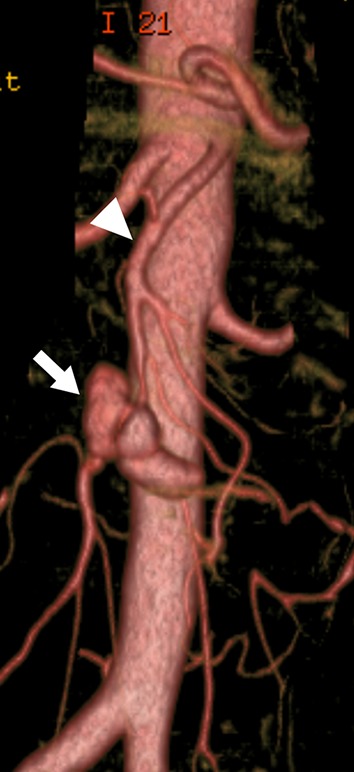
Three-dimensional volume rendered CT angiography shows an infected aneurysm (arrow) of the superior mesenteric artery (SMA) secondary to infectious endocarditis. Arrowhead shows the main trunk of the SMA
